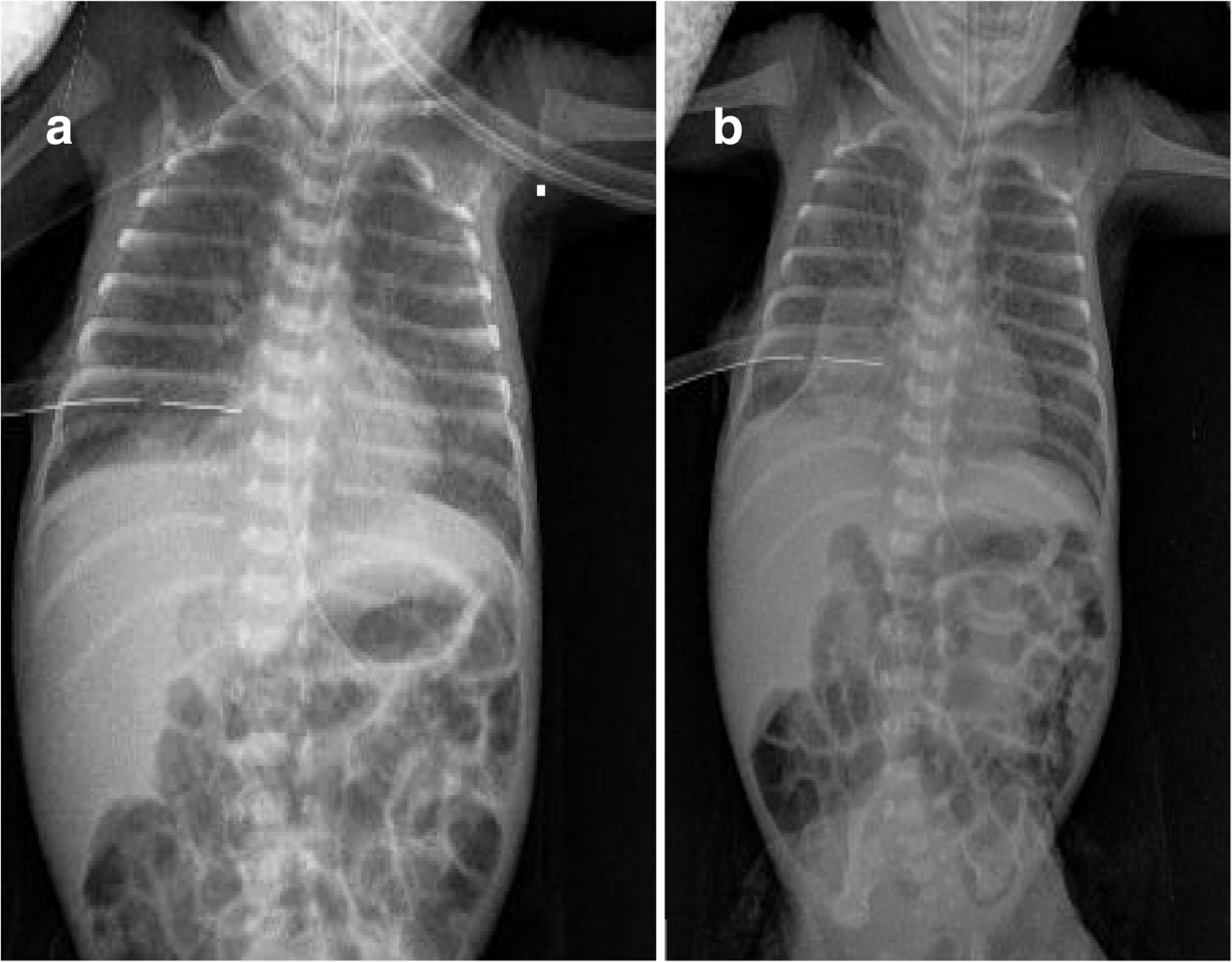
Chest x-ray after fibrin glue injection. Chest x-ray at 30 min (a) and 12 h (b) after fibrin glue injection. A minimal PTX was appreciable in (a) and disappeared in (b). At the distal tip of the chest tube is visible a radiopaque area referable to the fibrin glue clot more evident in (b) than in (a)
